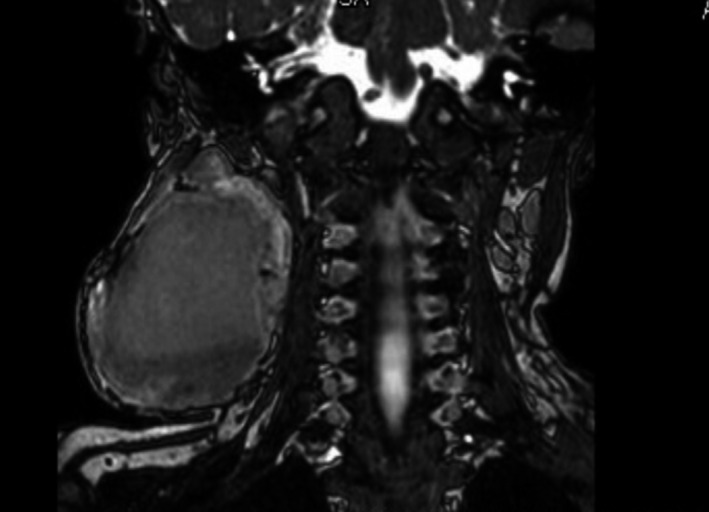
Magnetic resonance imaging indicating central cavitation of IH with peripheral hemosiderin, consistent with intralesion hemorrhage, at age 14 mo
